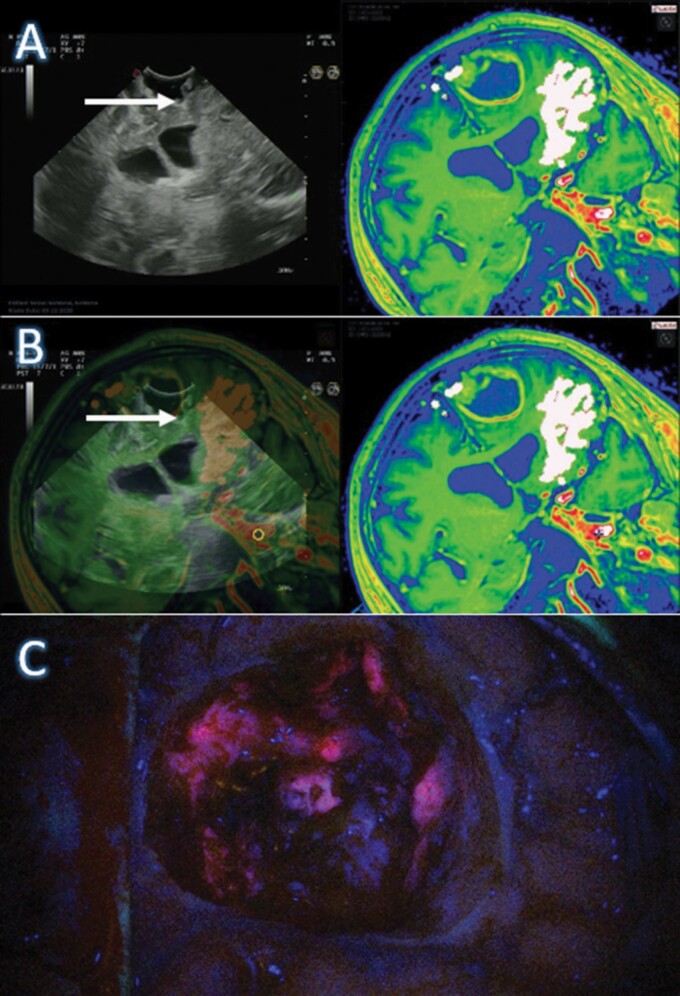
Integrated intraoperative ultrasound (US) and MRI (A and B) and 5-ALA in the surgical cavity (C) global assessment: fusion of MRI with integrated preoperative dissection of the fronto-aslant tract, intraoperative US, and 5-ALA to assess the extent of resection. *White Arrow*—contrast-enhancing tumor identified in the US-fMRI fusion and confirmed with 5-ALA fluorescence.
